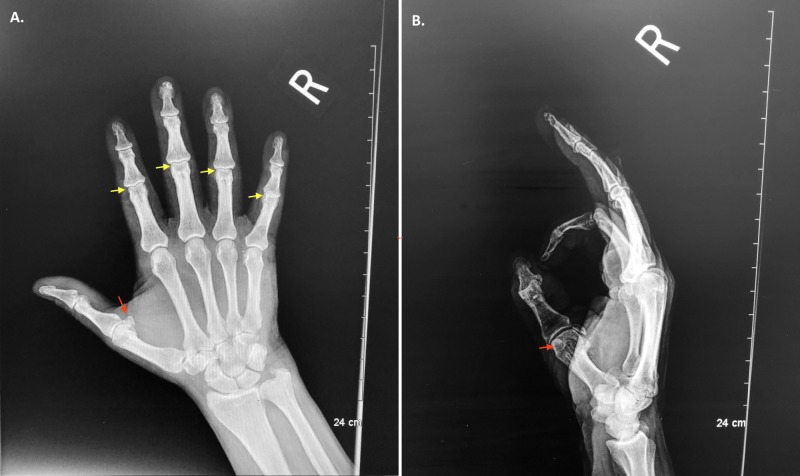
X-ray image of the right hand (two views) Findings: X-rays of the right hand demonstrate a mild hypertrophic change of the head of the first metacarpal (red arrow). Mild narrowing was evident at the proximal interphalangeal joints, with mild associated hypertrophic changes (yellow arrow). Impression: Mild early osteoarthritic changes; no evidence of fracture or dislocation
